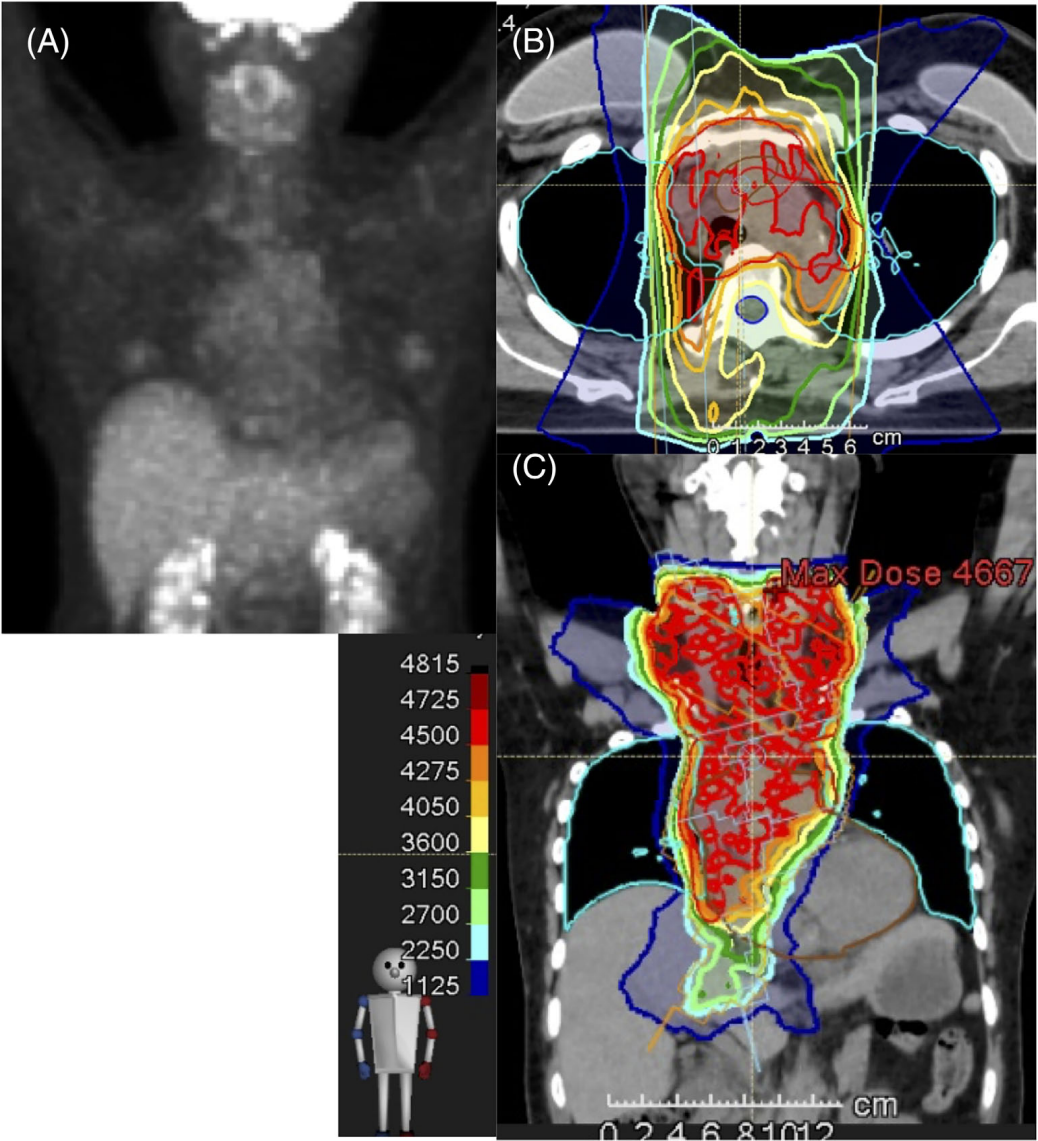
Positron emission tomography‐computed tomography (PET‐CT) images after two cycles of chemotherapy and dose distribution images of the volumetric modulated arc therapy (VMAT) plan. (A) Anterior view of the maximum intensity projection (MIP) image of the PET‐CT scan revealed little accumulation compared with that of pretreatment. (B, C) Transaxial and coronal dose distribution images and dose colour wash of the VMAT plan. V20, V5 and mean lung dose of the VMAT plan were 26.23%, 63.42% and 1403 cGy, respectively
